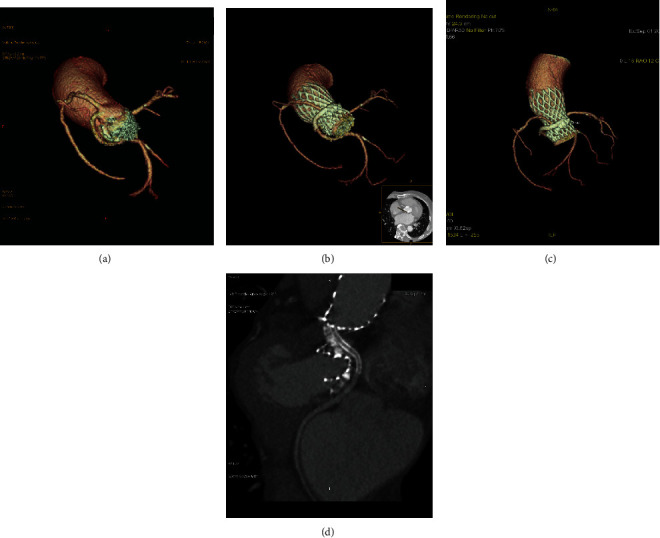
Six-month follow-up results after TAVR. (a) Cardiac computed tomography angiography showed a circumflex branch originating from right coronary sinus before TAVR. (b–d) Six-month follow-up after TAVR. CTA of the aortic and coronary regions suggested unobstructed coronary flow of the CX.
